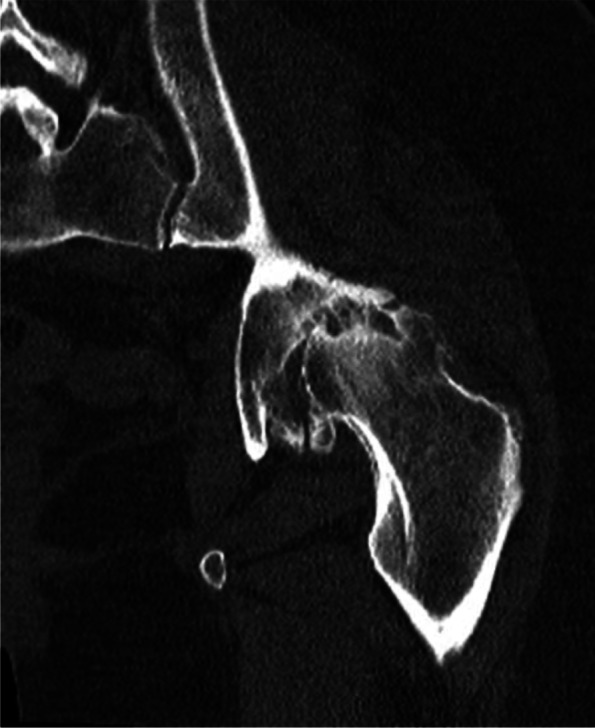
Sequence of the computed tomography of the pelvis which shows the acetabular retroversion combined with external rotation of the posterior pelvis and iliac crests
